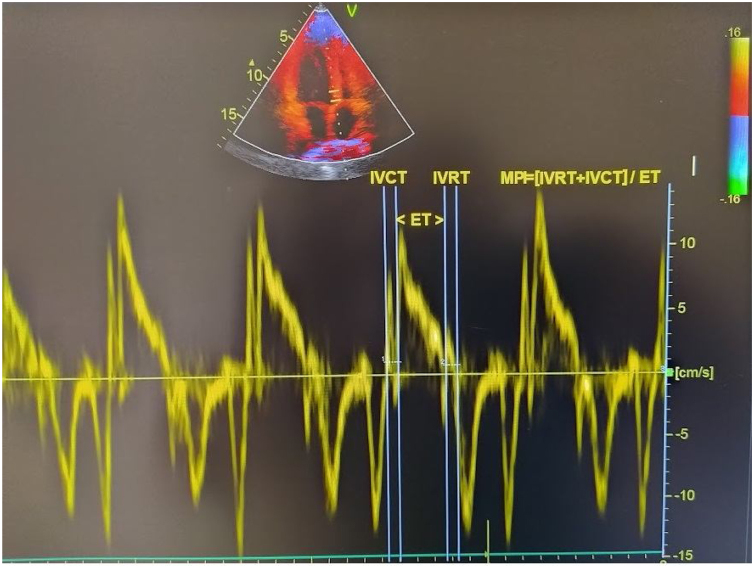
Tissue doppler-derived MPI.
